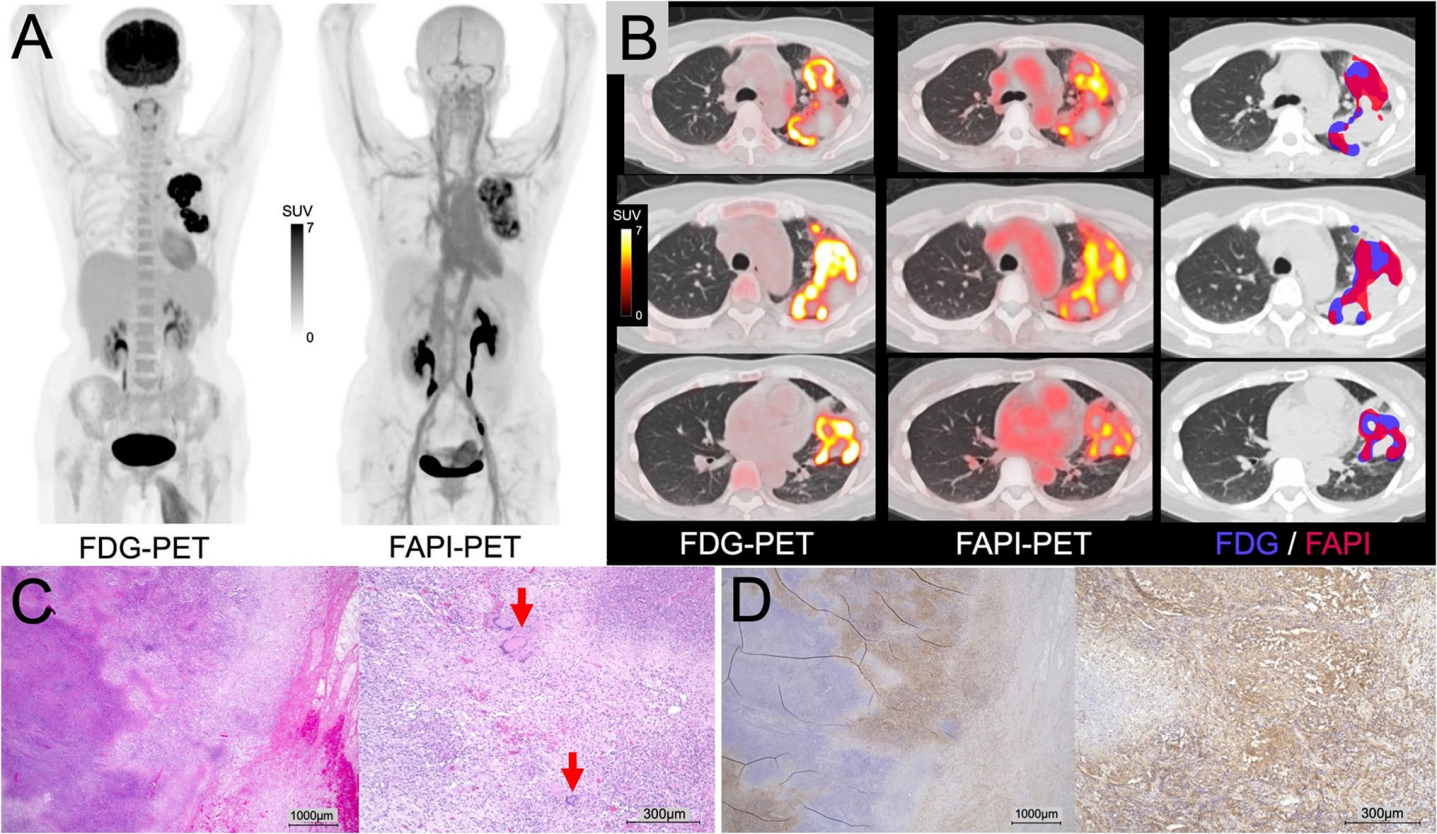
A 54-year-old woman suspected of lung cancer underwent [^18^F]FDG and [^18^F]FAPI-74 PET scans. CT revealed a 7-cm lesion in the left upper lobe. Despite detecting only necrotic cells on biopsy during bronchoscopy, malignancy was strongly suspected, leading to a planned lobectomy.
